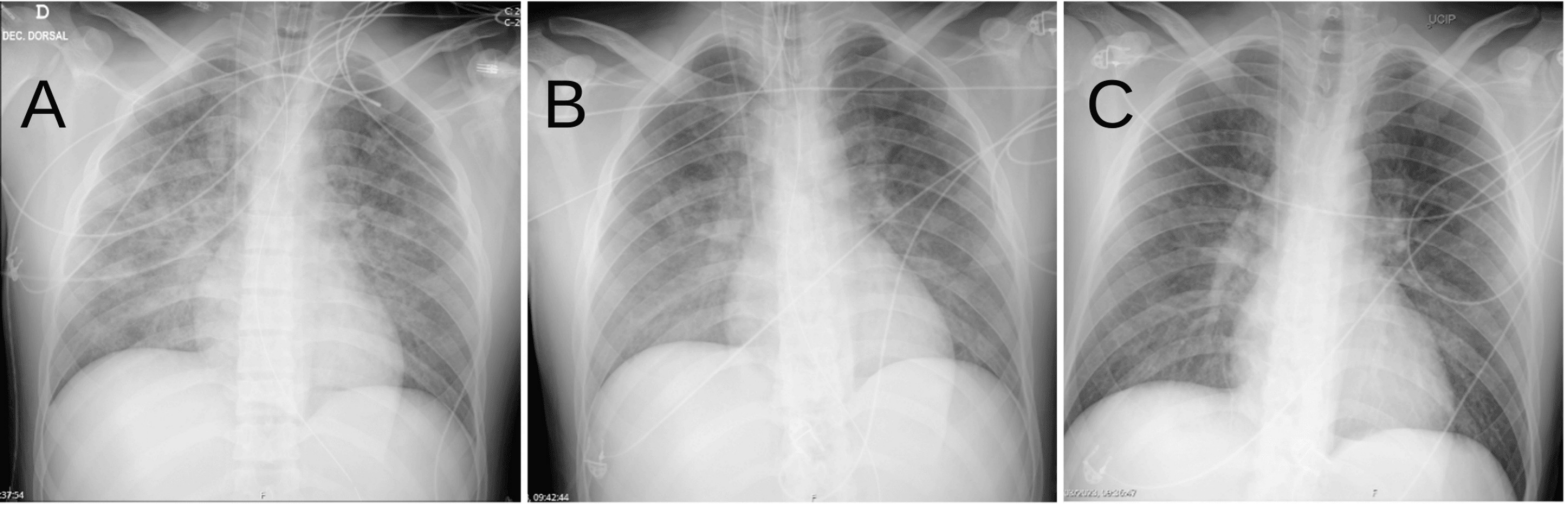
Evolution of chest X-ray at day one (A), day two (B), and day three (C) of intensive care unit stay
